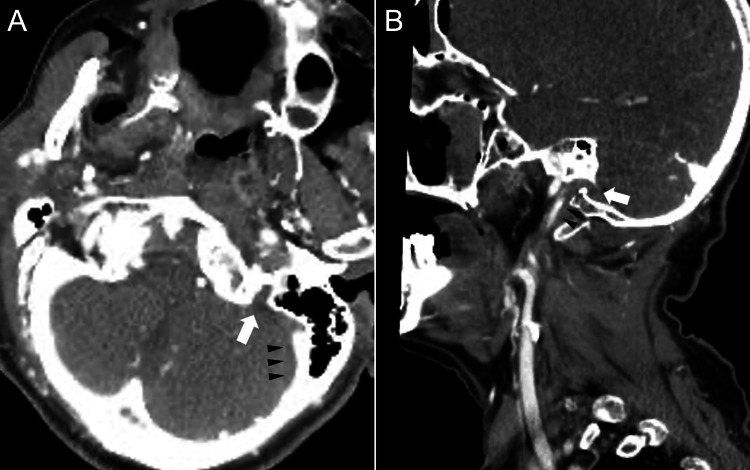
Computed tomography angiography on hospital day one (day of admission) in axial (A) and sagittal (B) views. White arrows indicate the left jugular foramen narrowed by the displaced fractured bone fragment in A and B. Black arrowheads in A indicate the sigmoid sinus with stagnant flow by the narrowed jugular foramen, while black arrowheads in B indicate the internal jugular vein.
